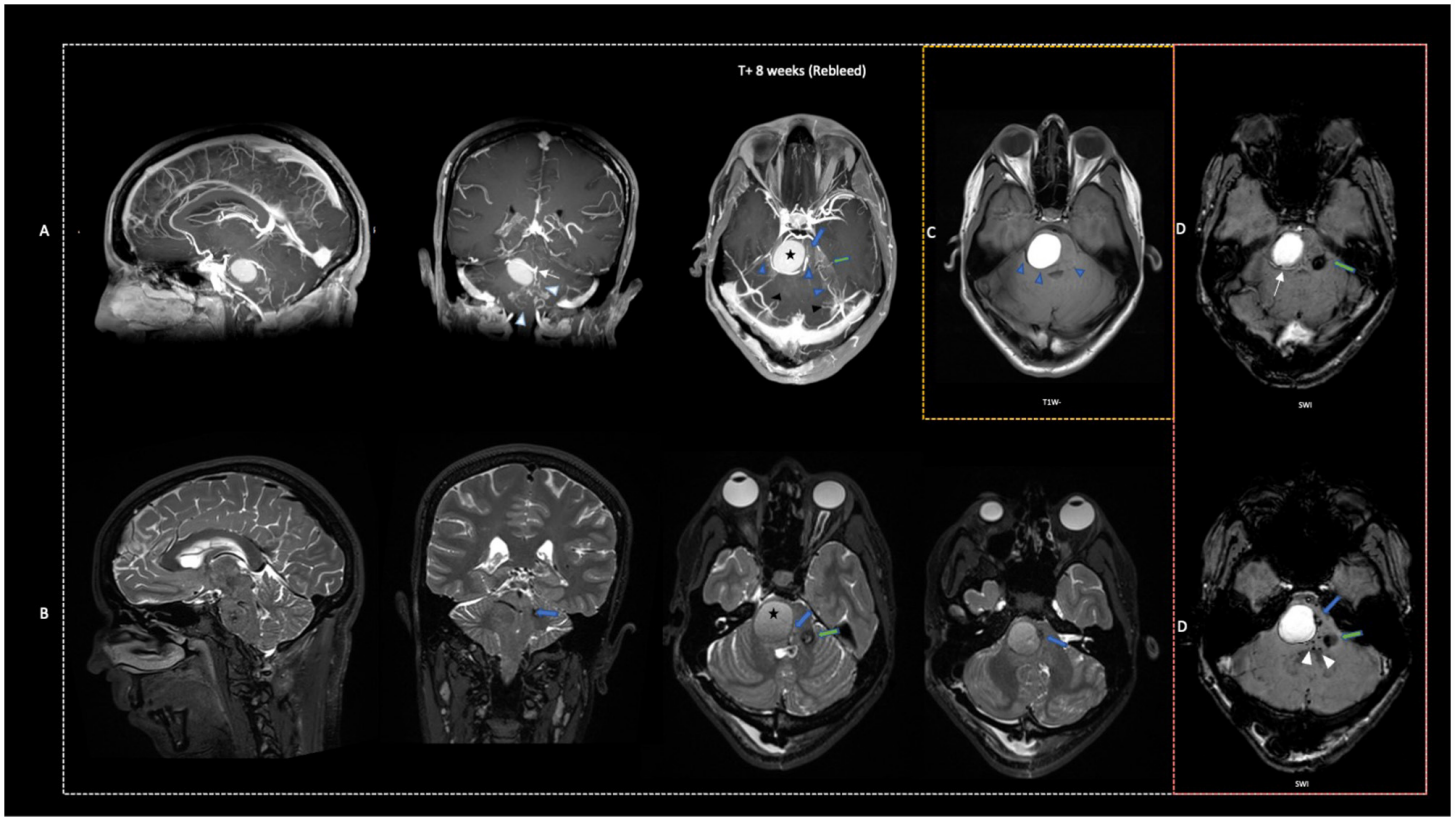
Rebleed after the first surgery. Sagittal, coronal, and axial MRI: (A) T1W + Gad; (B) T2W; axial only: (C) TW1 and (D) SWI. Showing late-stage subacute hematoma or extracellular meth-Hb (black star) with uniform hyperintense T1W signal and iso-hyperintense T2W signal. Target CCM (blue arrow) and untreated contralateral CCM (green arrow) seen as mixed signal intensity on T2W and blooming artifacts on SWI remain. Uniform filling of the main DVA draining vein but slightly stretched over hematoma as it drains into collecting vein on T1W+ (white arrow) and SWI (white arrow). Evidence of elevated pressure in the DVA system from the recurrence of prominent connecting channels (white arrowheads) on T1W+ and SWI.
